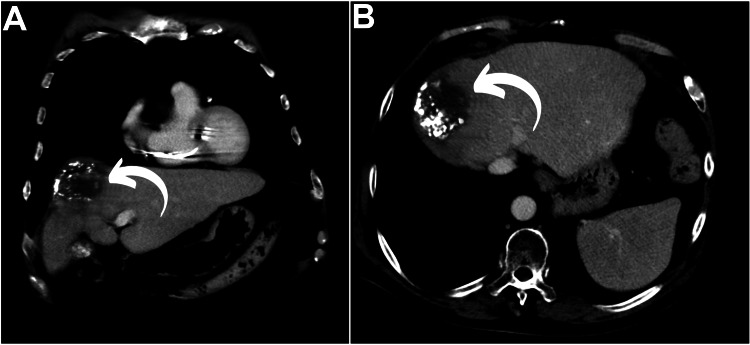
Follow-up abdominal CT (A, B) Partial tumor shrinkage and interval coalification were observed following transretinal chemoembolization (curved arrows).
